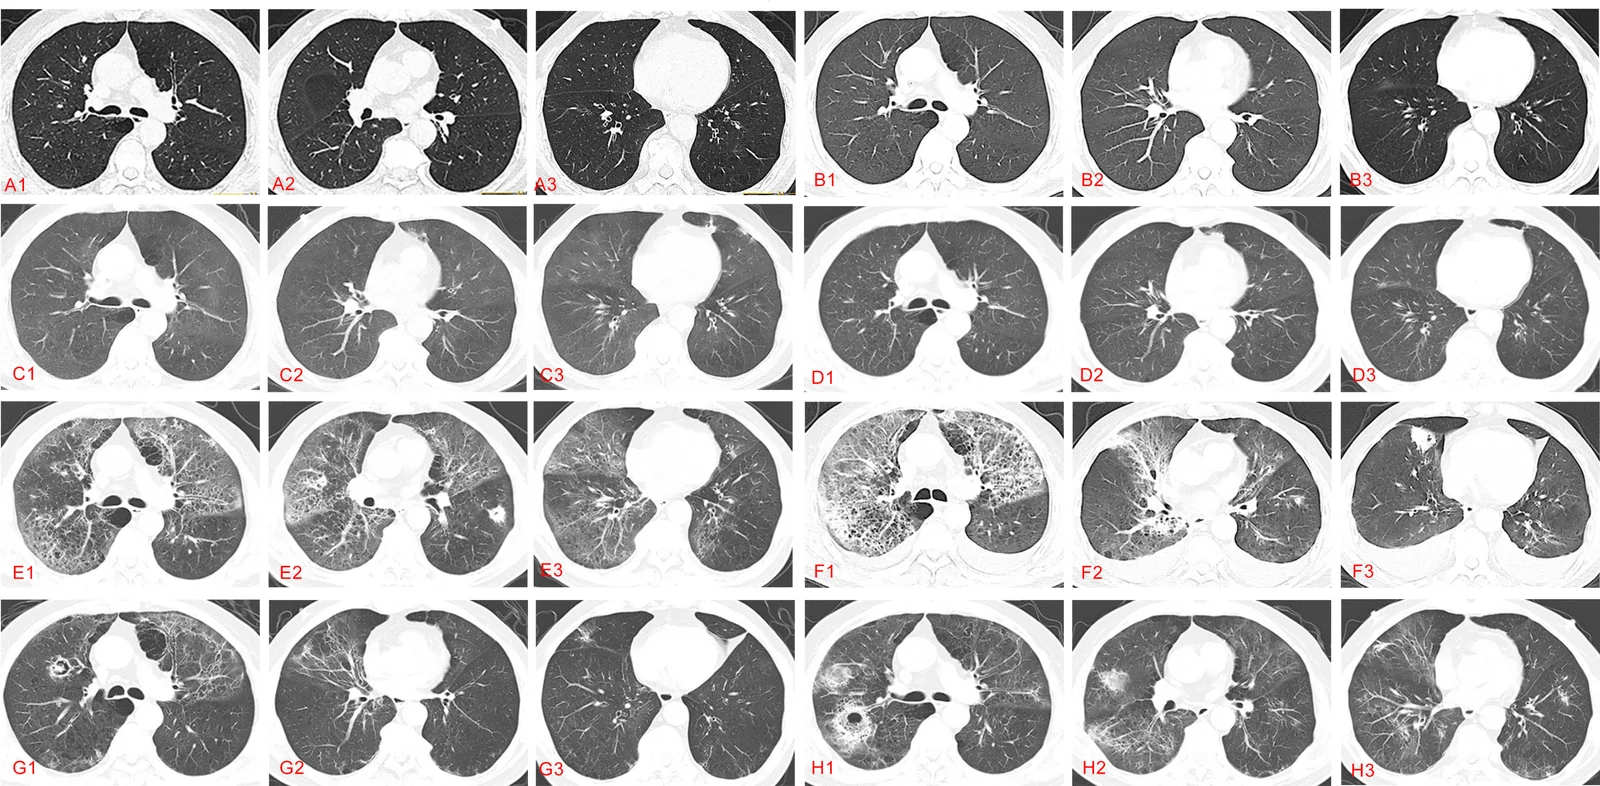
Changes in chest CT imaging. (A1–A3) Bilateral pulmonary emphysema. (B1–B3) Bilateral pulmonary emphysema. (C1–C3) Newly developed multiple large patchy slightly high-density opacities in both lungs. (D1–D3) After methylprednisolone treatment. (E1–E3) Reticular shadows. (F1–F3) Reticular shadows + solid density opacities. (G1–G3) After treatment with TMP/SMX + voriconazole. (H1–H3) Continuing TMP/SMX + voriconazole treatment. CT = computed tomography, TMP/SMX = trimethoprim-sulfamethoxazole.
